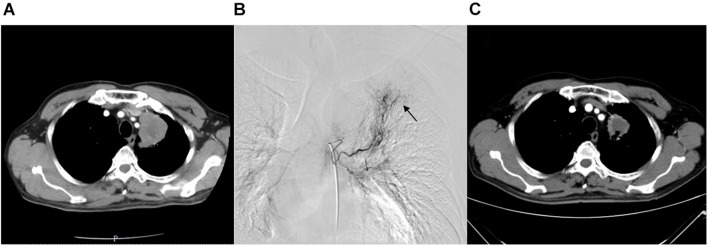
A 68-year-old female with advanced lung squamous carcinoma underwent the combination therapy of DEB-BACE and anlotinib. (A) Chest CT revealed a mass in the left lung superior lobe before treatment. (B) During the DEB-BACE procedure, the tumor was fed by the left bronchial artery (black arrow). (C) The patient achieved a PR after 1 month of combination therapy. DEB-BACE, drug-eluting bead bronchial arterial chemoembolization; CT, computed tomography; PR, partial response.
